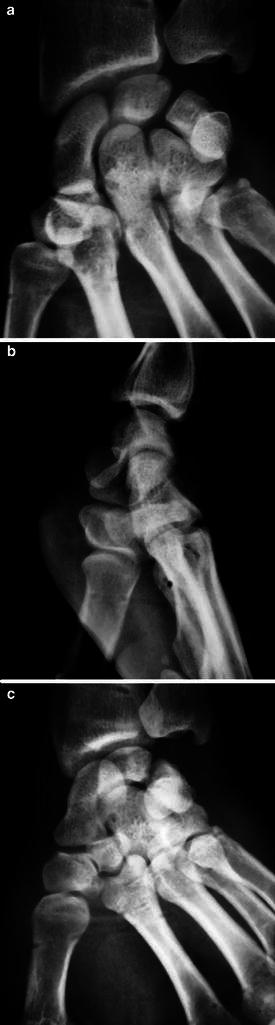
Four-week-postoperative radiographs (anteroposterior, lateral, and oblique views) show maintained carpometacarpal joints of the second, third, and fourth fingers after fixator removal
